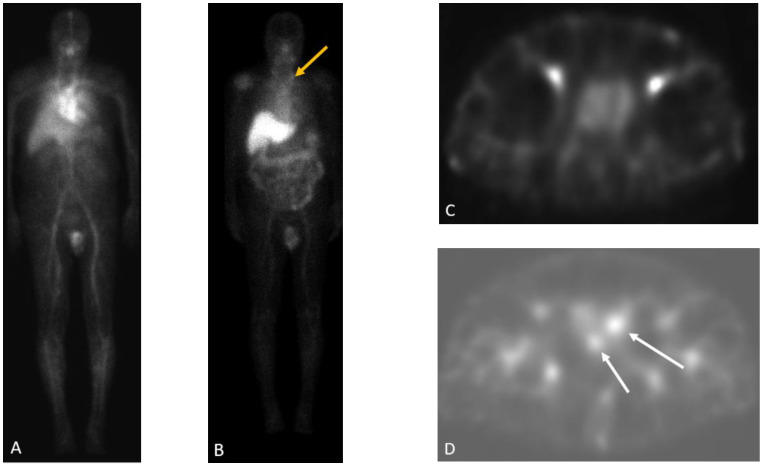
Sixty-four-year-old man with history radical prostatectomy for prostate cancer (Gleason 4 + 4) with rising PSA. In111 capromab pendetide whole body blood pool (A) and 96 h delayed (B) images demonstrate a focus of increasing radiotracer activity in the left neck base corresponding to a metastatic supraclavicular (Virchow) node (yellow arrow in B). SPECT blood pool (C) and delayed (D) images of the pelvis demonstrate two foci of increasing radiotracer activity in the right and left prostate bed, compatible with local recurrence (white arrows).
